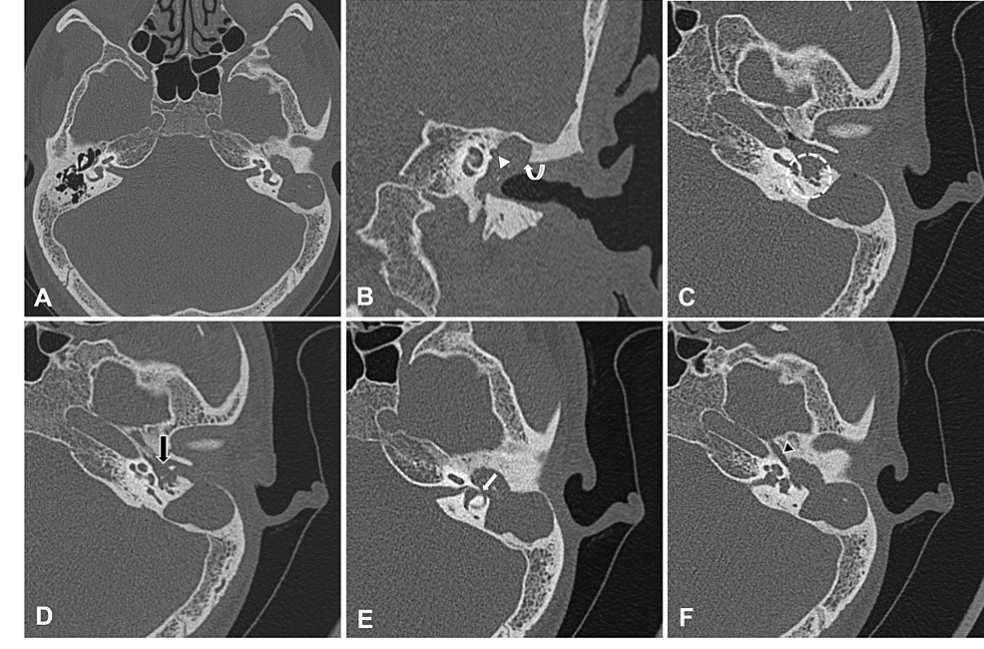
Axial HRCT image (A) showing a large well-defined soft tissue density in the left middle ear extending into the aditus and mastoid antrum, causing bony expansion and destruction of the malleus and incus with loss of ice cream cone appearance. Coronal reformatted CT image in the same patient (B) shows soft tissue density occupying the epitympanum, mesotympanum, and hypotympanum. It is seen causing erosion of the scutum (curved arrow) and inferior wall of the horizontal portion of the facial canal (arrowhead). Axial HRCT image (C) showing soft tissue (encircled) is seen extending into the sinus tympani and facial canal recesses. Axial HRCT image showing the destruction of stapes (D) and mastoid cortex dehiscence with thinning of dural sinus plate (D). Axial HRCT image (E) shows a focal defect in the wall of the lateral semicircular canal (white arrow) suggestive of perilymphatic fistula. Soft tissue extension into the eustachian tube (black arrowhead) was also seen (F). HRCT: high-resolution computed tomography
